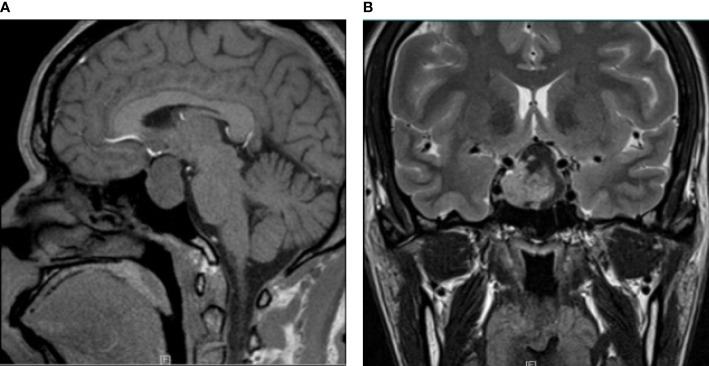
Radiological image of mixed gangliocytoma-pituitary adenoma. (A) Preoperative MRI shows a lesion with sagittal hypointensity on T1-weighted imaging. (B) T2-weighted coronal contrast imaging shows a hyperintensity signal with a prominent waist sign. The tumor passed the tangent of the medial aspects of the intracavernous and supracavernous internal carotid arteries, but did not extend beyond the intercarotid line.
